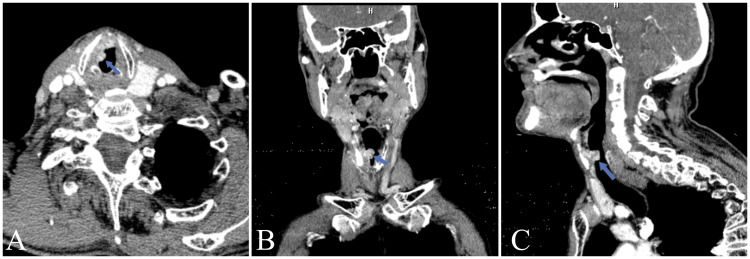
Axial (A), coronal (B), and sagittal (C) images of contrast-enhanced neck CT Originating from the contiguity of the thyroid cartilage at the right glottic level, advancing in the submucosal area, narrowing the laryngeal air column medially, extending to the supraglottic level superiorly, removing the fatty plane between the thyroid cartilage is the mass with a lobulated contour that contains hyperdense areas of calcification and extends anteriorly to the anterior commissure. It is a heterogeneous mass lesion that is 20×11×10 mm in size (AP×TR×CC) with no significant contrast enhancement observed (blue arrows). AP: Anteroposterior, TR: Transverse, CC: Craniocaudal
